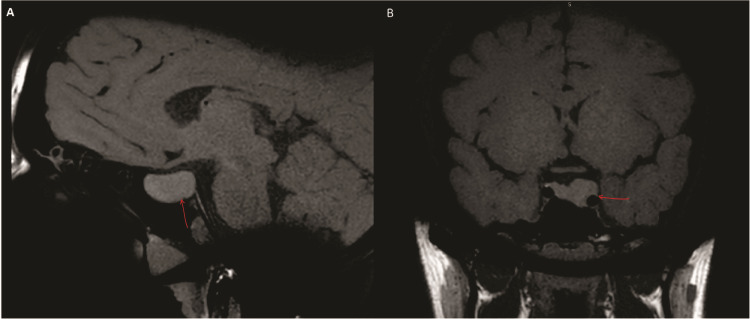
Sagittal and coronal T1-weighted images Sagittal T1-weighted image showing a 1.7 x 1.5 x 2 cm pituitary macroadenoma. The pituitary stalk and remaining pituitary gland are deviated to the right (A). T1-weighted coronal image showing the same macroadenoma invading the cavernous sinus and wrapping around the internal cerebral artery (B).
